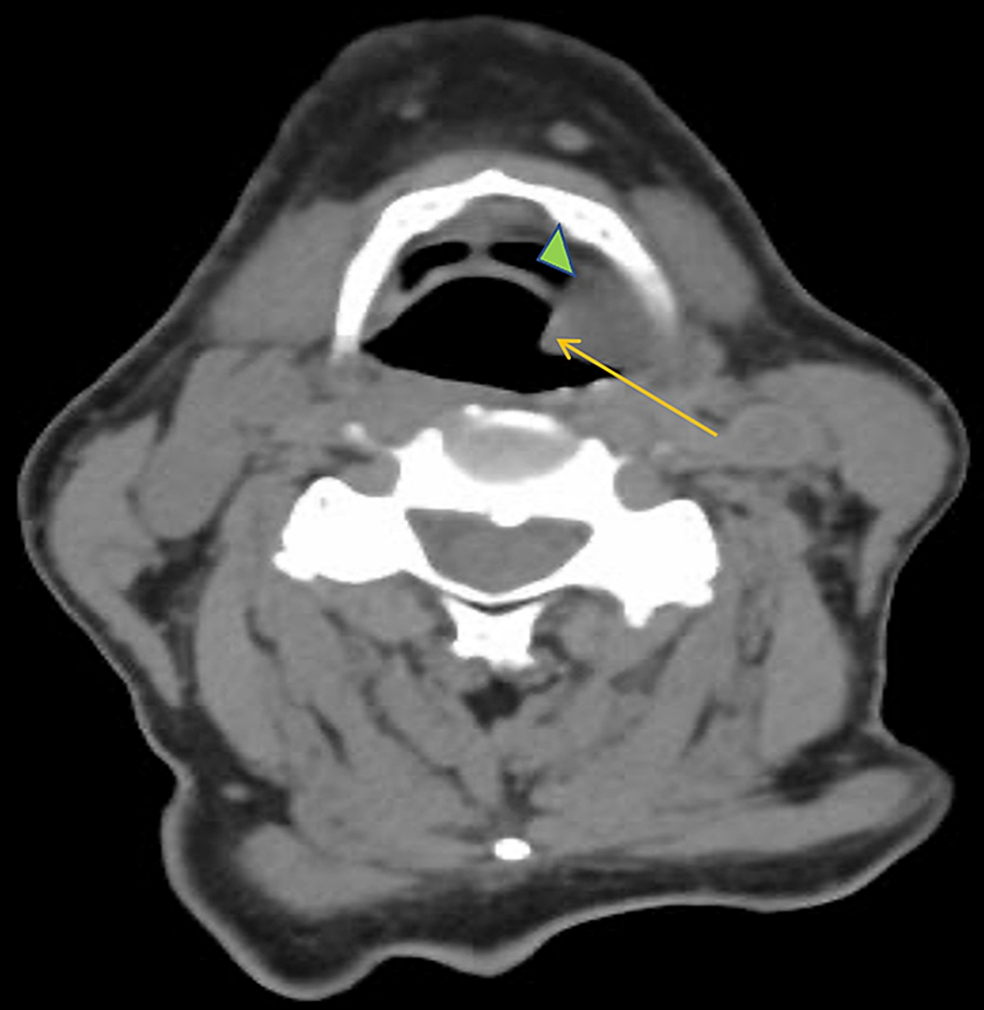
CT scan of the neck showed a soft tissue mass (arrow) on the left piriform sinus and vallecula (arrowhead) extending inferiorly to the false vocal cords (out of view).
